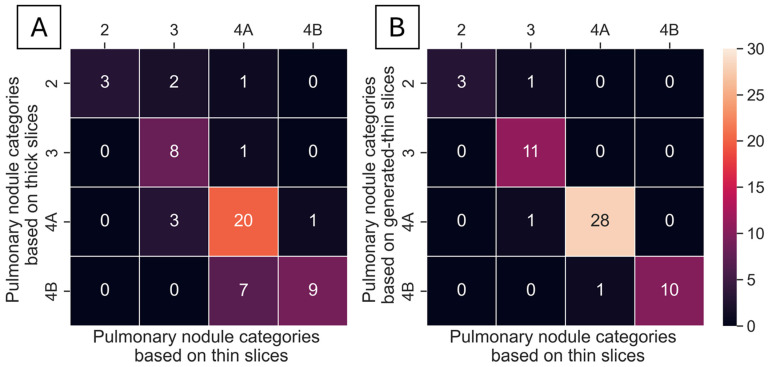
Confusion matrix based on Lung-RADS v2022 lung nodule classification for (A) thick-slice–thin-slice CT image pair and (B) generated-thin-slice–thin-slice CT image pair.
